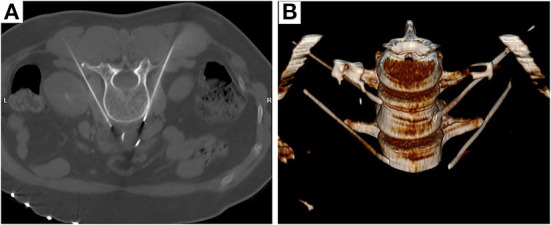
Radiofrequency targeting points (A) and three-dimensional reconstruction (B) of CT-guided thoracic sympathetic nerve radiofrequency thermocoagulation using the L3 segment as an example.
